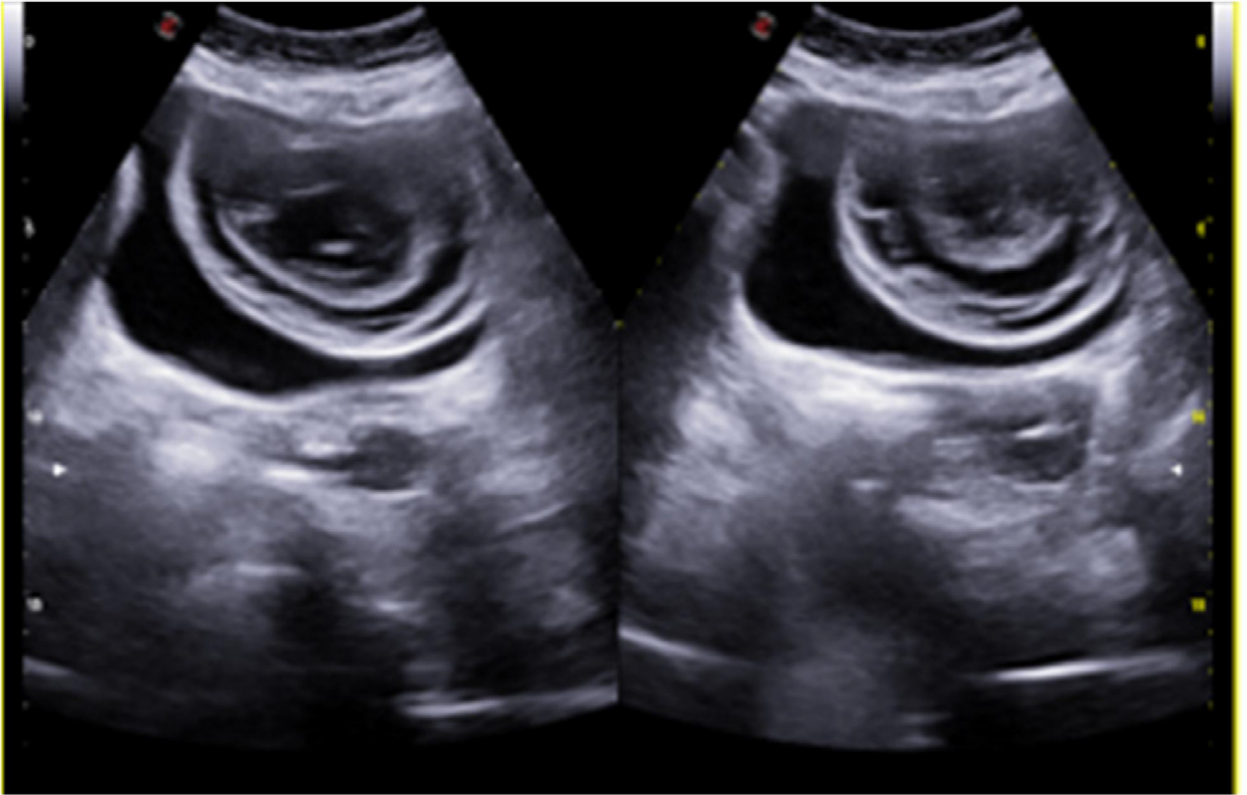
Abdominal ultrasound: Echogenic lesion of approximately 8 cm apparently adhered to the superior bladder wall, with a target-shaped morphology.
